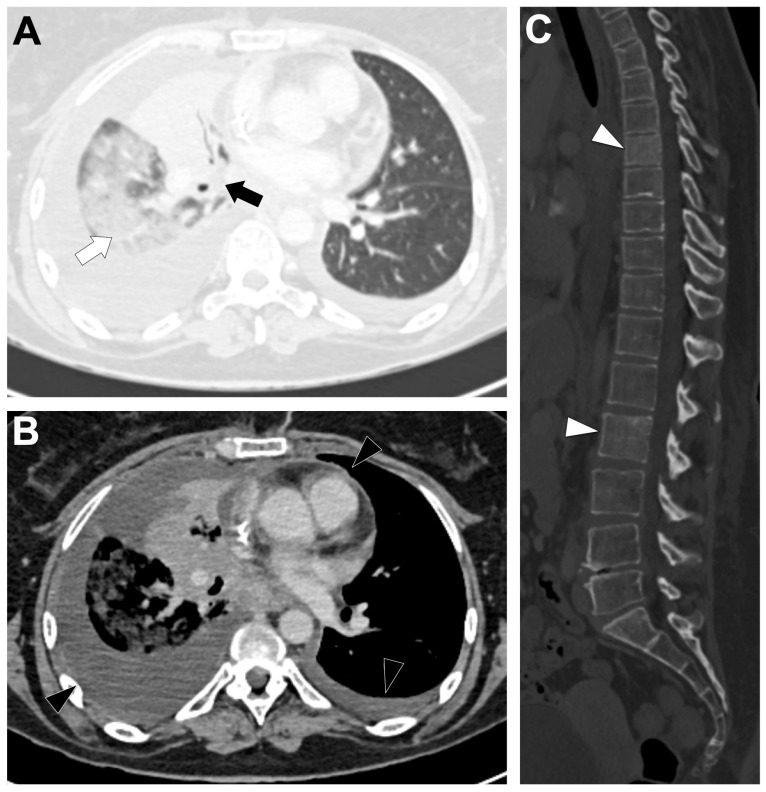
Representative baseline CT imaging findings in patients with BRAF exon 15 V600E (class 1) substitution lung adenocarcinoma (LUAD). A 43-year-old woman with a heavy smoking history presented with a central right upper lobe tumor (black arrow) causing segmental atelectasis and surrounded by ground-glass opacity (white arrow) (A). Bilateral pleural and pericardial effusions with metastatic spread were observed (black arrowheads) (B). On bone-window imaging, sclerotic vertebral metastases were identified (white arrowheads) (C).
